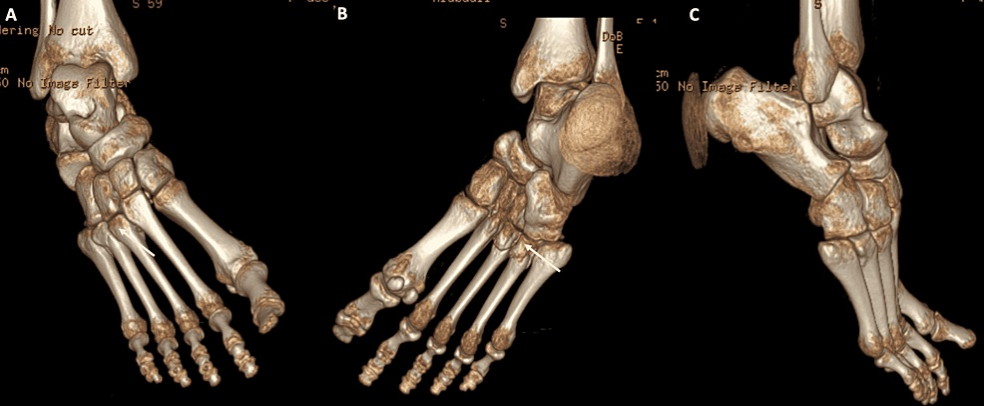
A 3D-reconstructed image of the computed tomography (CT) scan that was done to the right foot (A) Dorsal surface of the right foot. (B) Plantar surface of the right foot. (C) Lateral aspect of the right foot.
